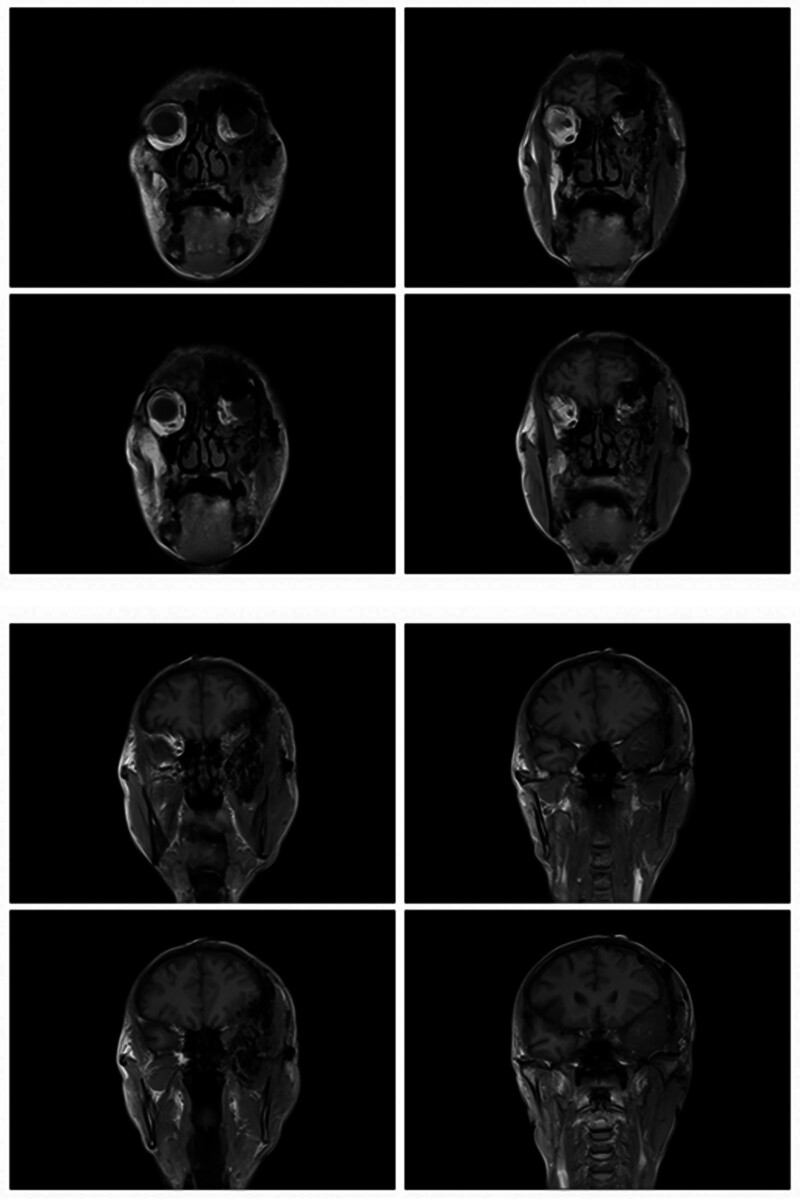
Patient postoperative MRI coronal T1 phase. MRI = magnetic resonance imaging.
